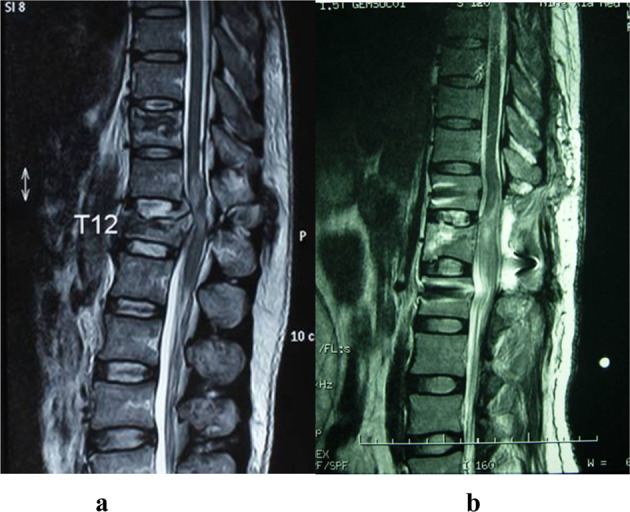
Typical mid-sagittal views of MRI images of the patients in G1 and G2, showing the focal or extensive long T2 signals in the spinal cord. a Male 25 yrs, from G1, focal long T2 signal was found only at the spine trauma level, showed limited the cord lesion. b Female, 40 years-old, from G2 L1 burst fracture, a typical T10 SCI, with long T2 signals found at the injury level extended to the tip of the cord, indicating the wide range of cord injury
